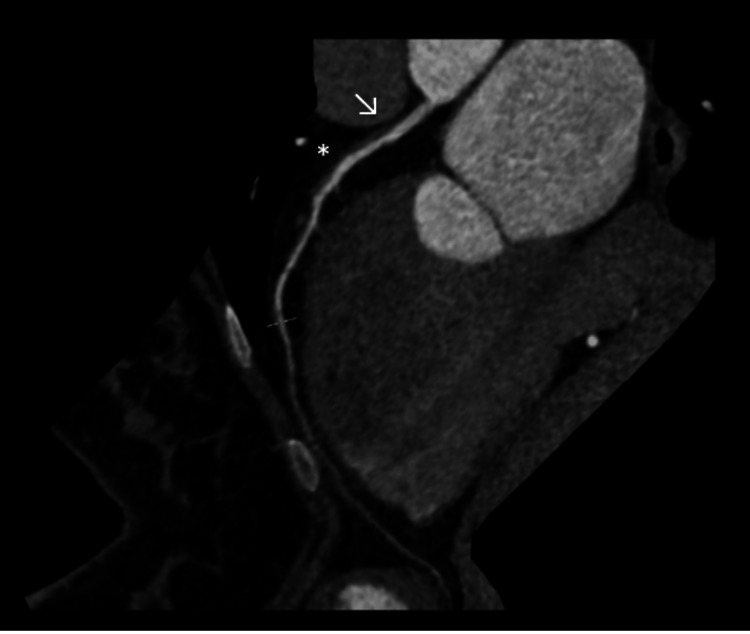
CT coronary angiogram images showing dissection involving the left main stem and proximal left anterior descending artery. An eccentric, long-segment linear hypodensity with associated wall haziness is seen in the left main stem artery (arrow), extending into the proximal LAD (asterisk).
